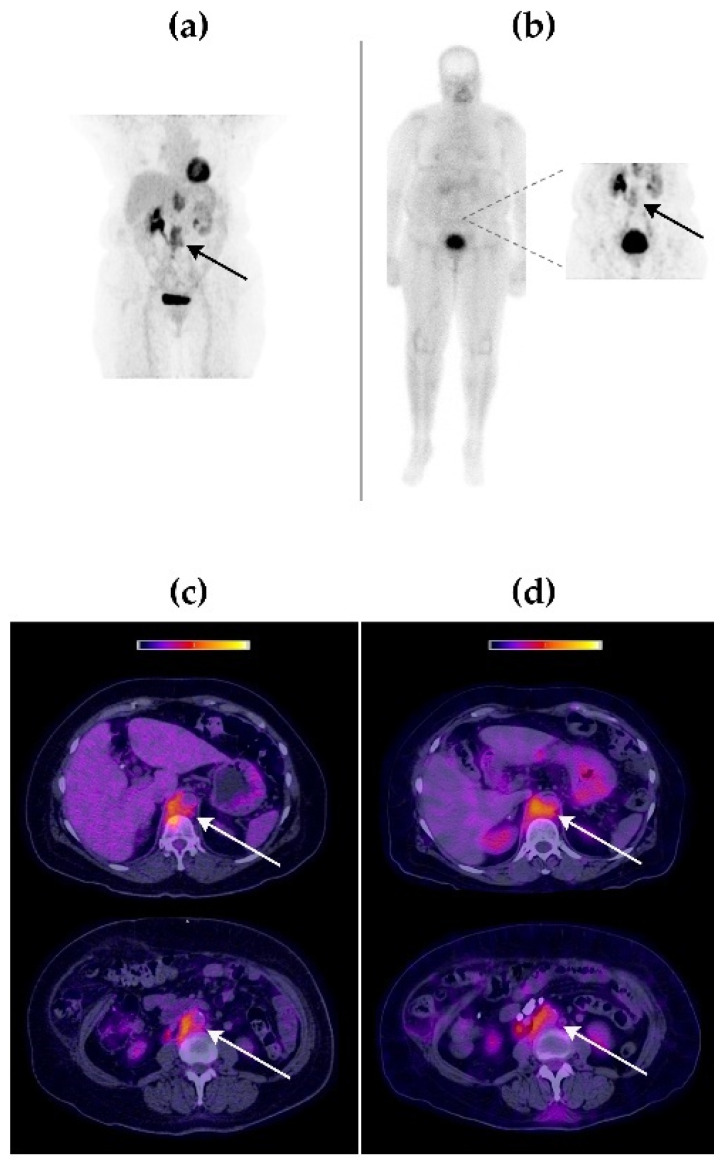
Patient with cervical squamous cell carcinoma and retroperitoneal lymph node recurrence (Patient 3). (a) Maximum-intensity projection [^18^F]FDG PET image at 1 h p.i. (b) [^99m^Tc]Tc-iFAP whole-body planar scintigraphy in anterior projection. Inset: abdominal SPECT at 3 h p.i. (c) [^18^F]FDG PET/CT axial sections. (d) [^99m^Tc]Tc-iFAP SPECT/CT axial sections. Lymph node conglomerates at the right retrocrural and retroperitoneal intercaval-aortic level with retrocaval extension. No recurrent primary lesion was observed at the pelvic level. The uptake of both radiopharmaceuticals was concordant in all tumor lesions.
